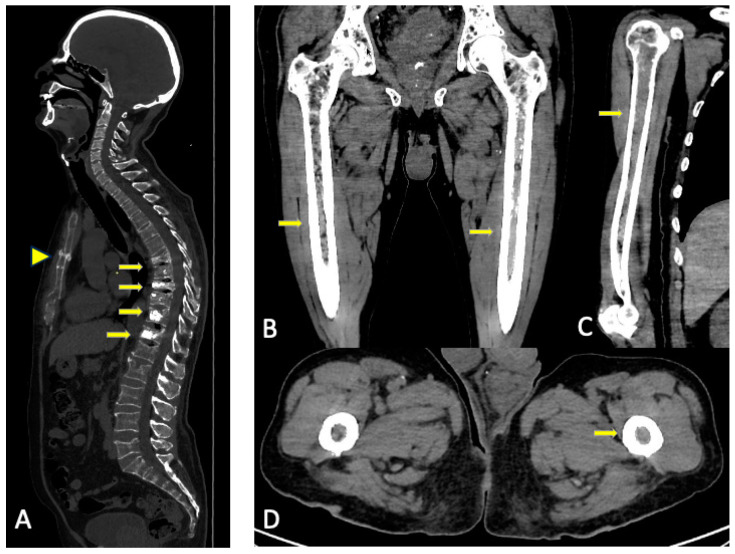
Conventional CT multiplanar reconstructions. (A) Reconstruction in the sagittal plane for better assessment of the spine and the sternum. Multiple fractures in the thoracic and lumbar spine, some of them with vertebroplasty (arrows) with no visible lytic lesions. Horizontal fracture in the sternal body (arrowhead). (B–D) Reconstruction in different planes of the long bones for better assessment of cortical and the medullar cavity. Substitution of fatty bone marrow for areas of soft tissue density without endosteal scalloping in both femora and right humeri (arrows). The asymmetrical distribution favors tumoral infiltration over red marrow reconversion.
